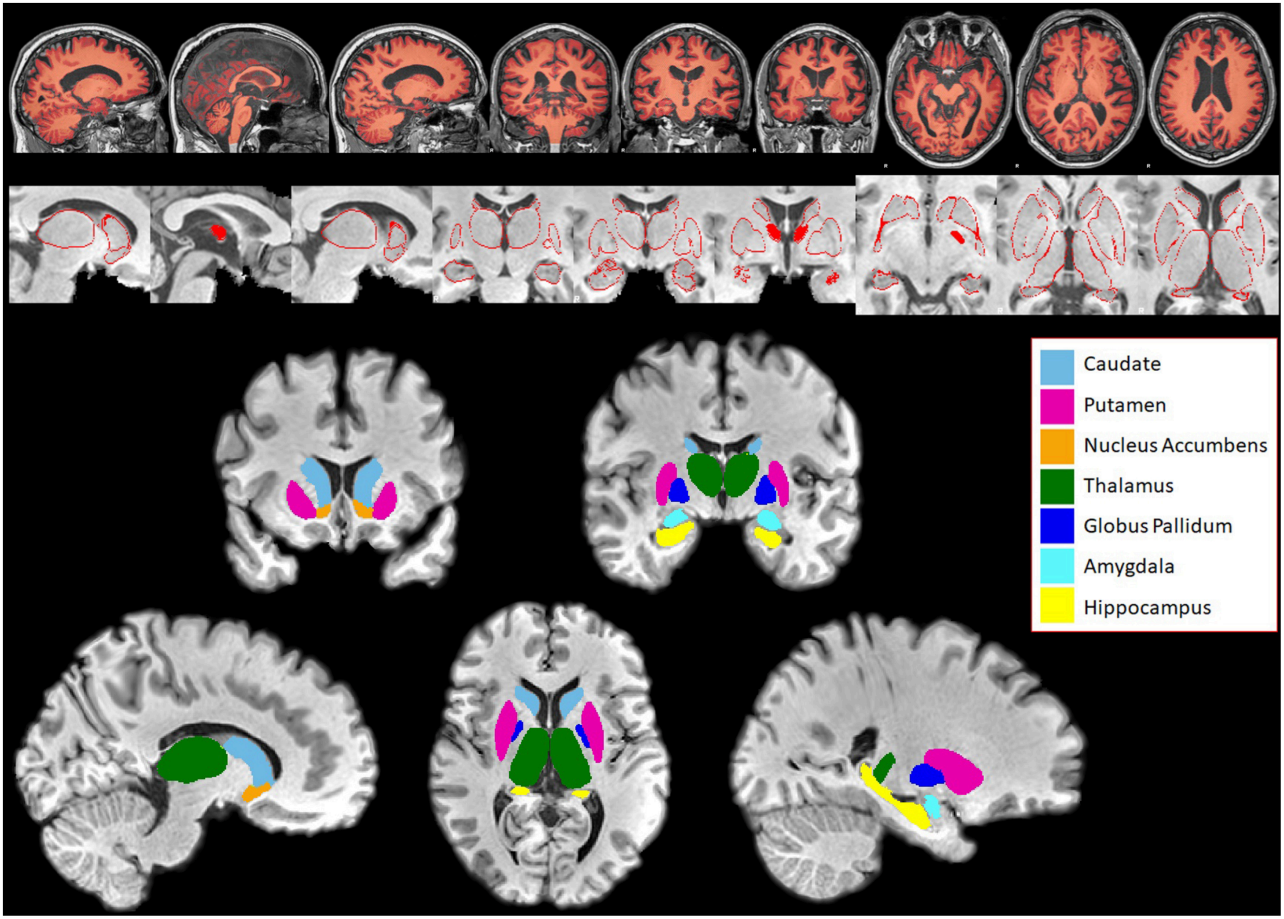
Subcortical structures segmented in Patients and Controls for comparison using FIRST. Top panel: Segmentation of white and gray matter generated by SIENAX overlaid on a subject's brain. Middle panel: Series of pictures of red outlines that represent segmented subcortical structures overlaid on top of the subject's T1-weighted brain. These pictures are examined for quality assessment purposes. Bottom panel: Subcortical structures segmented using FIRST, overlaid on top of the subject's skull stripped T1 weighted brain. Bilateral caudate, putamen, nucleus accumbens, thalamus, globus pallidus, amygdala, and hippocampus were segmented in both patients and controls.
